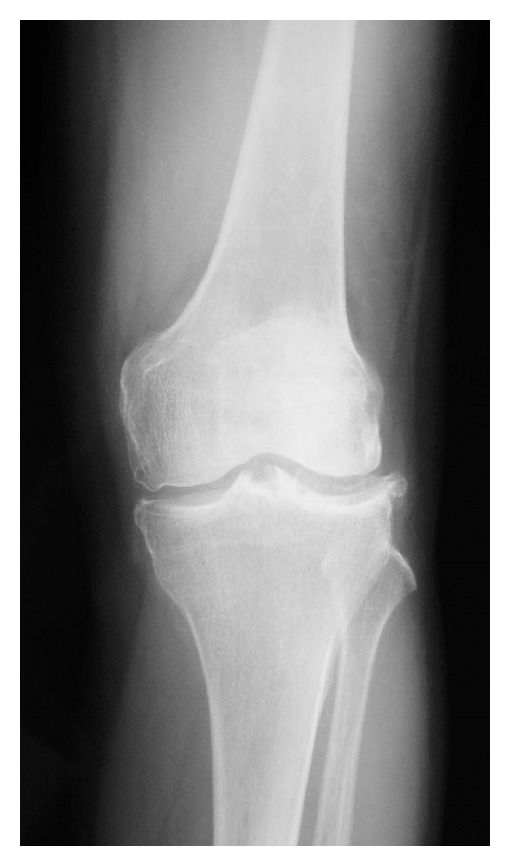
Radiograph of Case 2. AP view shows grade 3 lateral-dominant osteoarthritis according to Kellgren and Lawrence scale.
